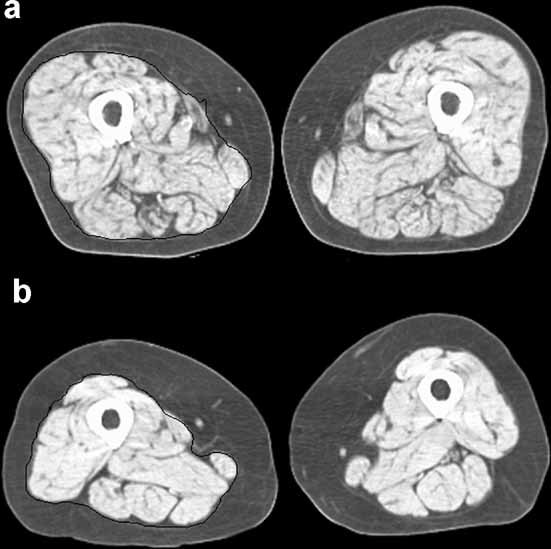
Representative CT images of the midthigh showing in black the outline of the region of interest encompassing the thigh muscle bundle used for area and attenuation measurements in this study. (a) Axial image showing extensive fatty infiltration of the muscle and having a thigh muscle lean tissue attenuation coefficient of 26 HU. (b) Axial image with a thigh muscle lean tissue attenuation coefficient of 38.6 HU.
